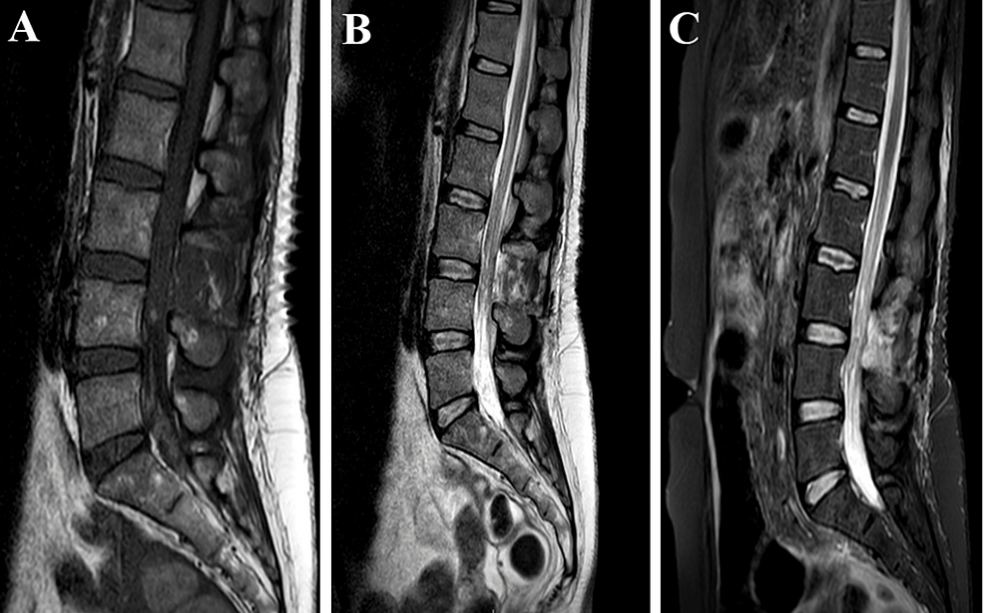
Immediate postoperative MRI after the decompression and removal of the lesion A: Sagittal T1-weighted MRI of the spine. B: Sagittal T2-weighted MRI of the spine. C: Sagittal STIR MRI of the spine. MRI: magnetic resonance imaging, STIR: short-TI inversion recovery
